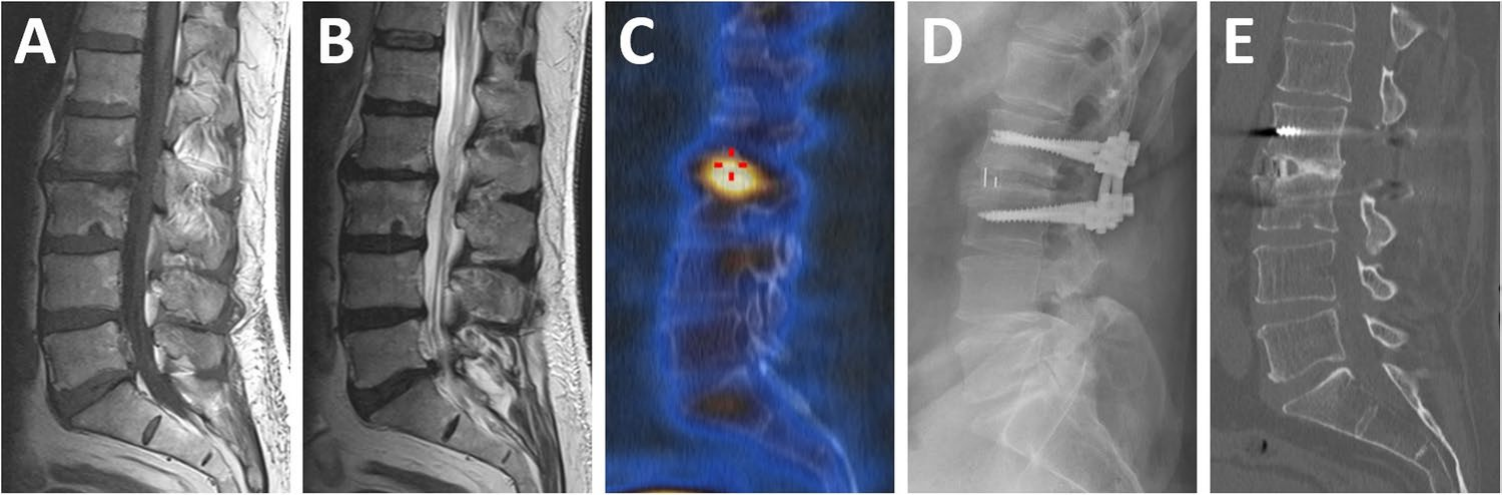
Multi-level degeneration (patient 30) seen on sagittal lumbar spine images. A T1W MRI, B T2W MRI, C SPECT/CT showing higher uptake in L2/3 level, D X-ray 6 months after surgery, E CT scan 2 years after surgery with fusion of the L2/3 intervertebral space
